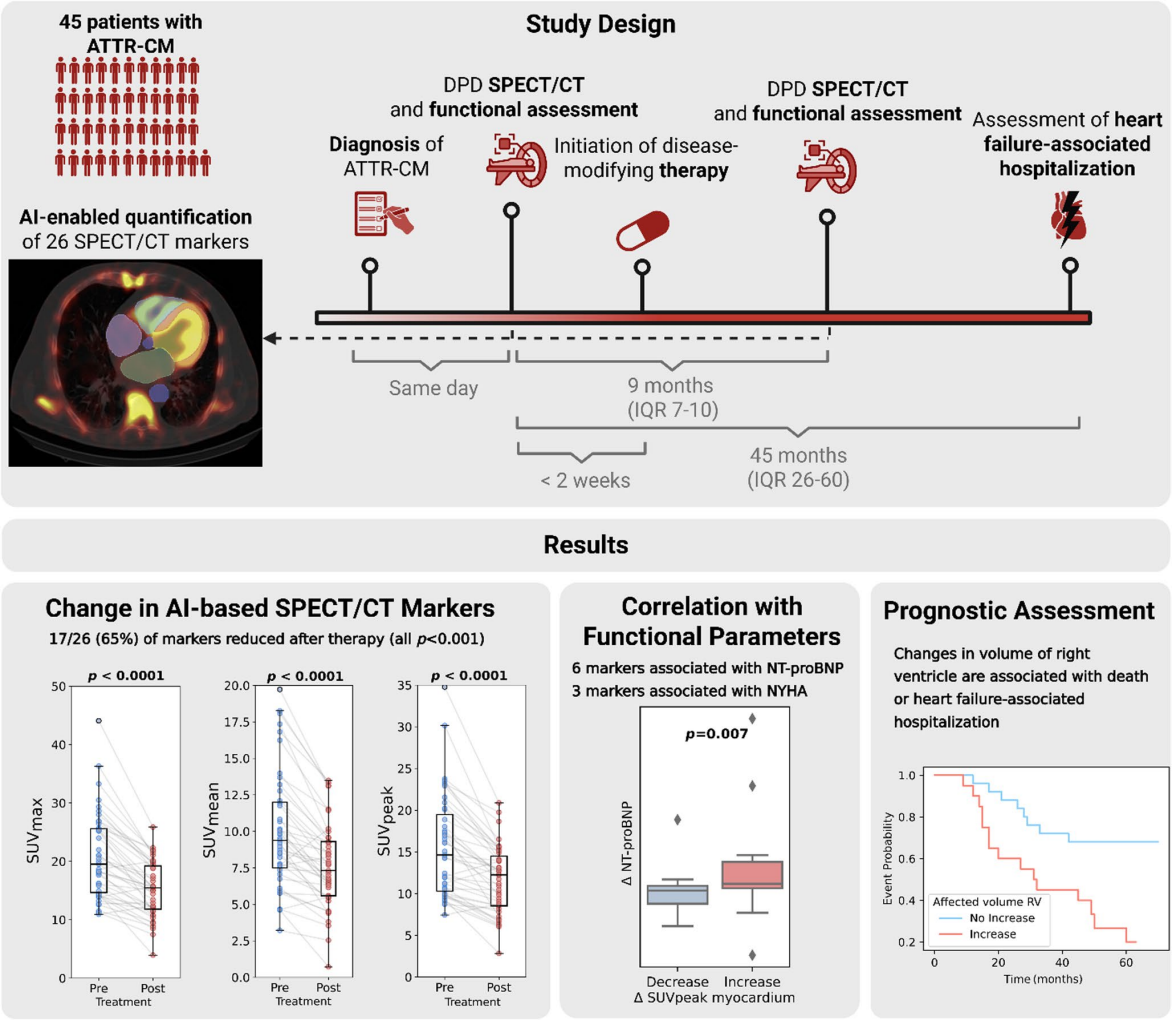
Graphical abstract detailing the design and main results of the study. Reduction in artificial intelligence (AI) assessed SPECT/CT markers after versus before treatment was associated with functional parameters and clinical outcomes
